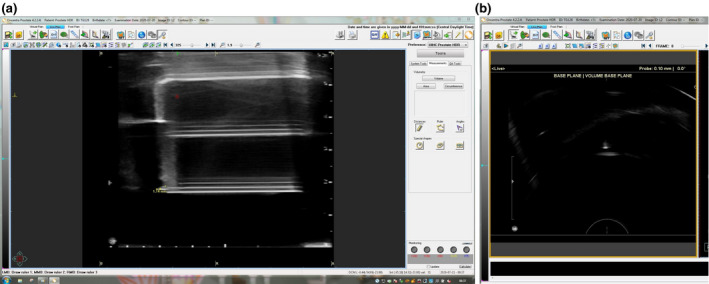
Sagittal (left) and transverse (right) ultrasound images of the ultrasound marker. The baseplane was set in the sagittal view within the center of the marker directed toward the ultrasound probe. Note that the probe depth is the depth of the transverse crystal, which is beyond where the baseplane was set. Upon setting the baseplane, and retracting the ultrasound probe, the depth of the brightest signal from the marker in the transverse plane, shown in the upper right‐hand corner of (right), was compared to the base plane position set in the sagittal view, which was 0.00 mm by definition
